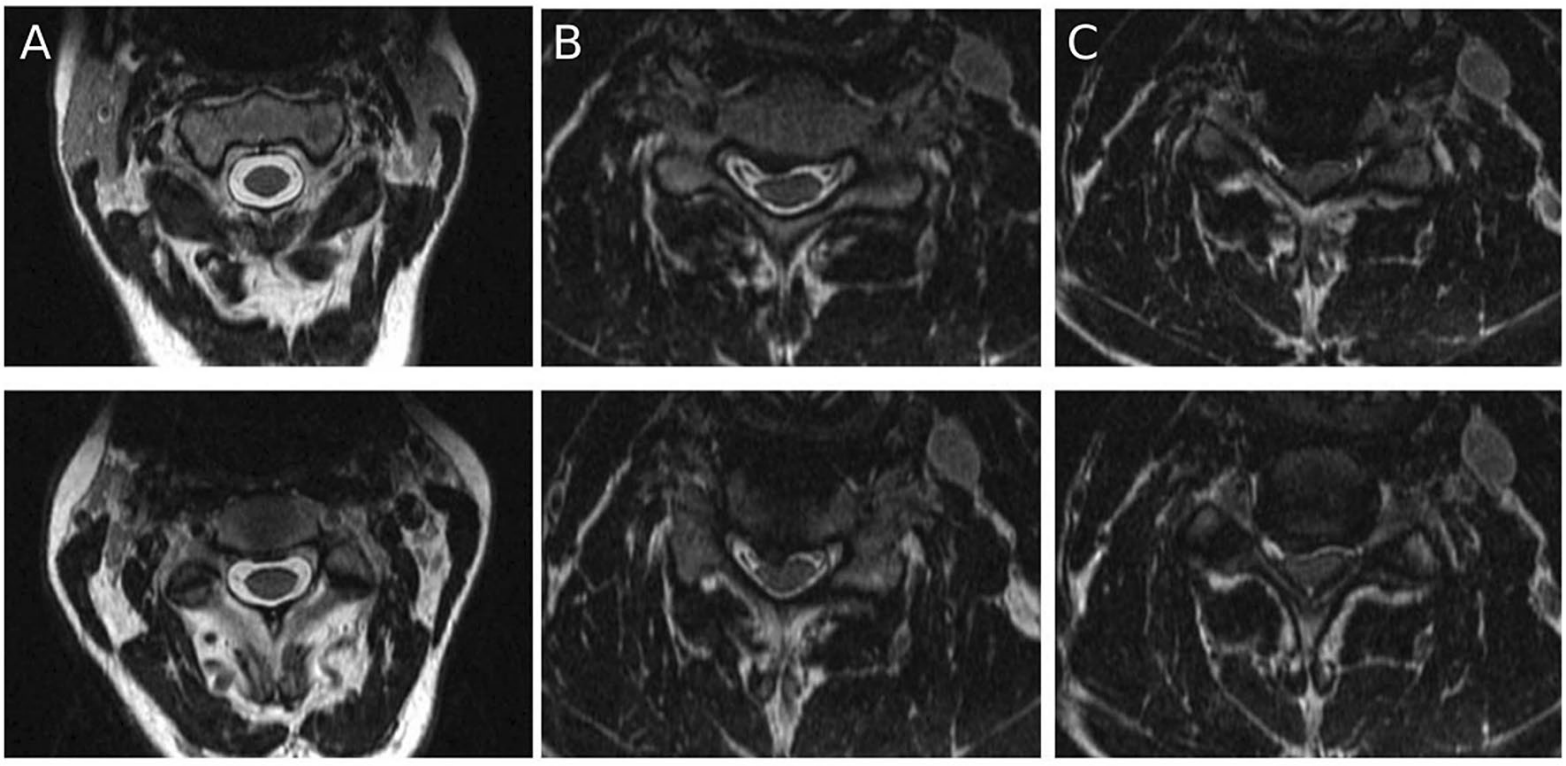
Representative axial T2-weighted MRI images showing (A) no spinal cord compression, (B) partial spinal cord compression, and (C) circumferential spinal cord compression.
